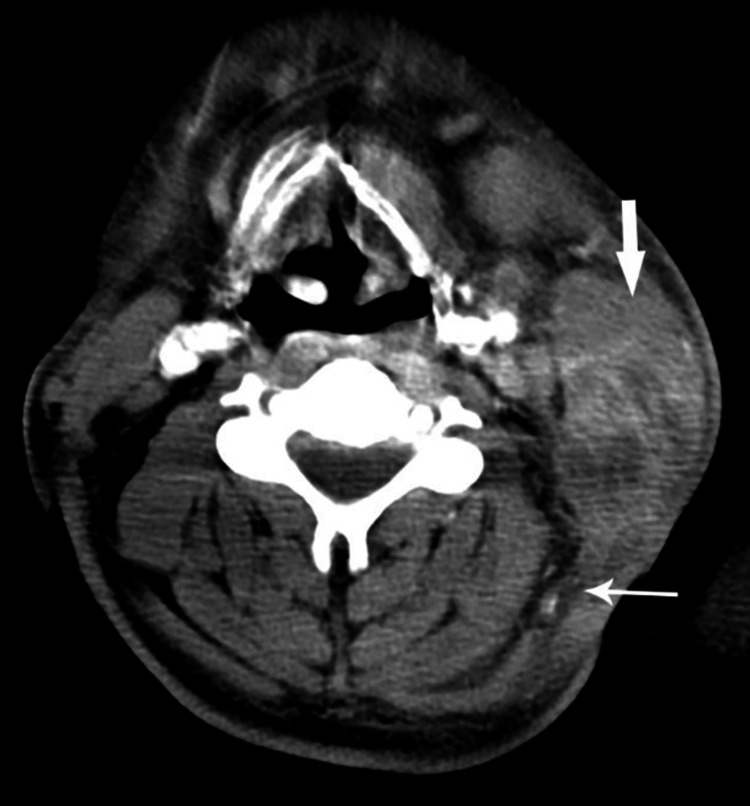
CT scan of the neck shows thickening and enhancement of deep cervical fascia (fasciitis) (thin arrow) enlargement and heterogenous enhancement of muscles (myositis) (thick arrow).
